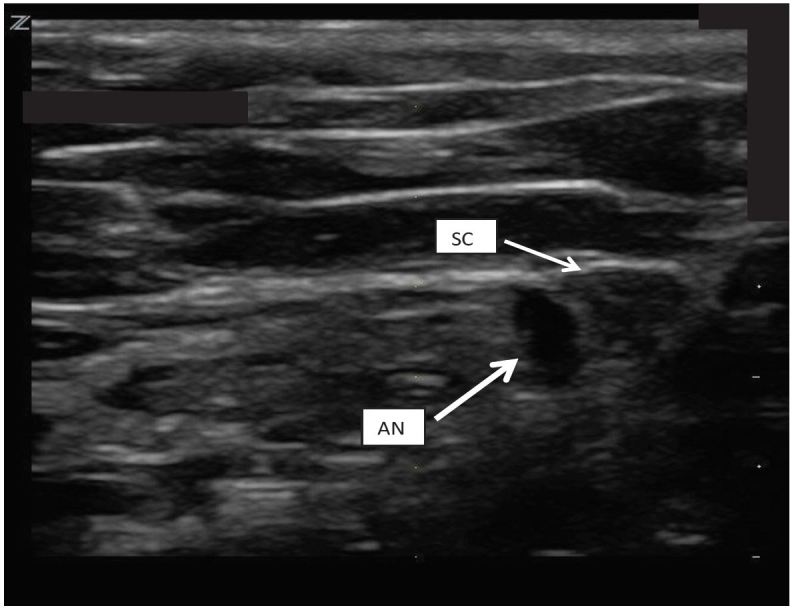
Coronal view. Sonographic anatomy of the spermatic cord (SC) and anesthesia (AN) solution deposited adjacent to the cord.
